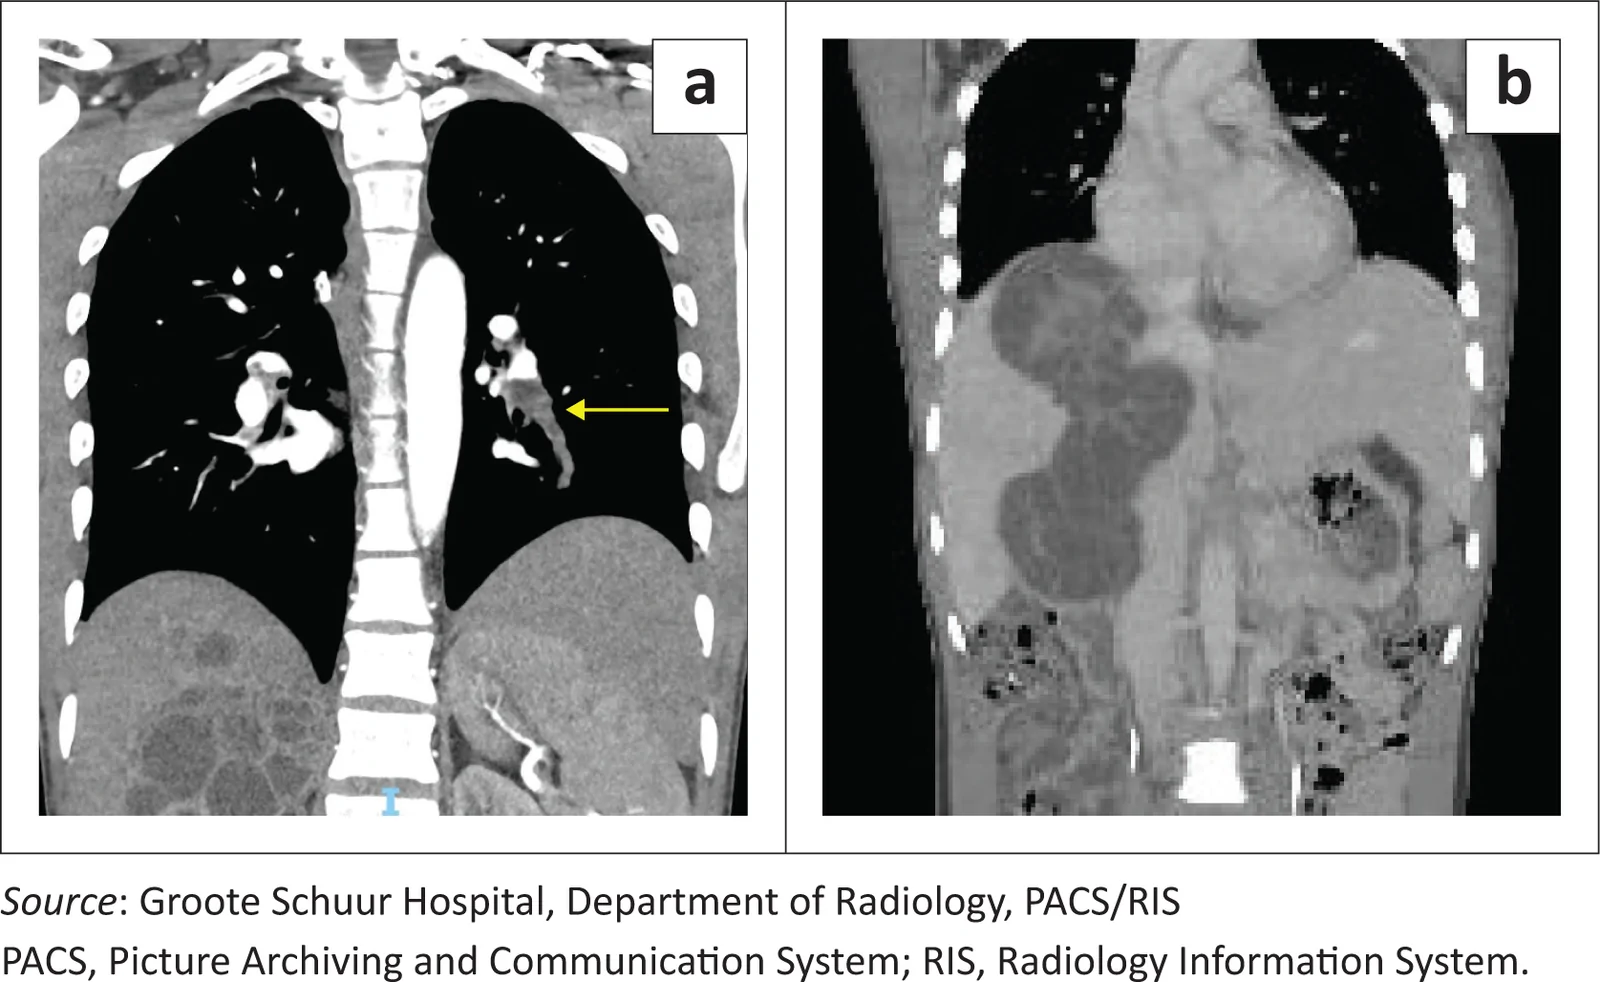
Contrast-enhanced CT images of a 24-year-old HIV-negative male who presented with a clinical picture suggesting pulmonary embolism. A contrast-enhanced CT showed multiple emboli in the right and left lower lobes (arrow) (a) and cysts in segments 4a and 8 between the inferior vena cava and the hepatic veins with suspected cyst invasion into the middle hepatic vein (b).
